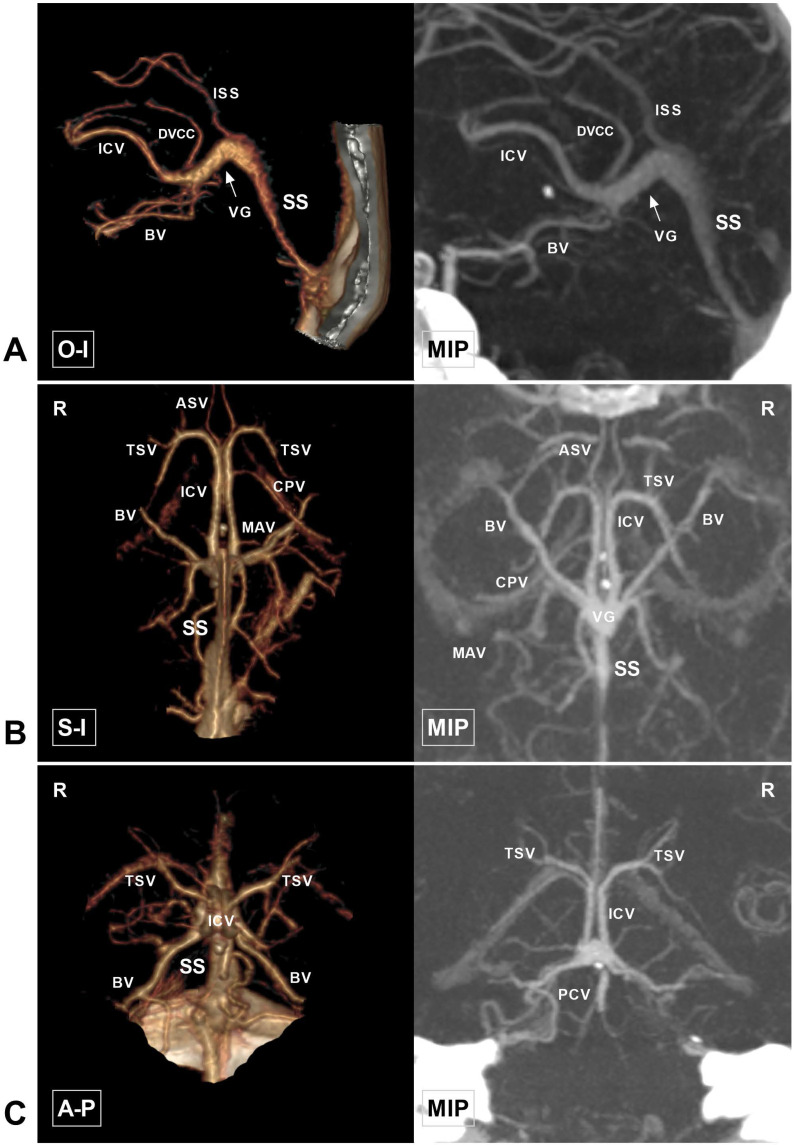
Anatomy of the cerebral deep vein on CTA. A, CTA and MIP in the O-I view show that the ICV extends backward as the VG (arrow). The VG continues as the SS and empties into the torcular herophili. The DVCC and ISS drain into the VG at their beginning and terminal portions, respectively. B, CTA and MIP in the S-I view show that TSV and ASV merge into the ICV. The bilateral ICVs and BVs merge into the VG and continue as the SS. The CPV and MAV draining into the ICV are also visible. C, CTA and MIP in the A-P view show that the TSV continues as the ICV and then merges with the BV. The PCV draining to the VG is visible. Abbreviations: A-P, anterior to posterior; ASV, anterior septal vein; BV, basal vein; CPV, choroid plexus vein; CTA, computed tomography angiography; DVCC, dorsal vein of the corpus callosum; ICV, internal cerebral vein; ISS, inferior sagittal sinus; MAV, medial atrial vein; MIP, maximum intensity projection; O-I, outside to inside; PCV, precentral cerebellar vein; R, right; S-I, superior to inferior; SS, straight sinus; TSV, thalamostriate vein; VG, vein of Galen.
